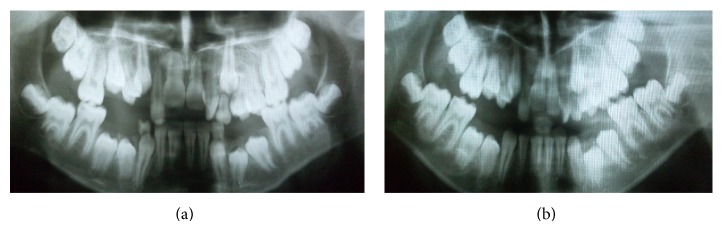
(a, b) Six-month and 12-month follow-up radiographs.
